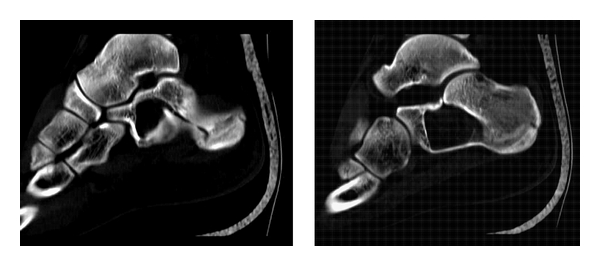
CT scan views on the sagittal plane showing an undisplaced pathologic fracture over a simple calcaneal cyst.
